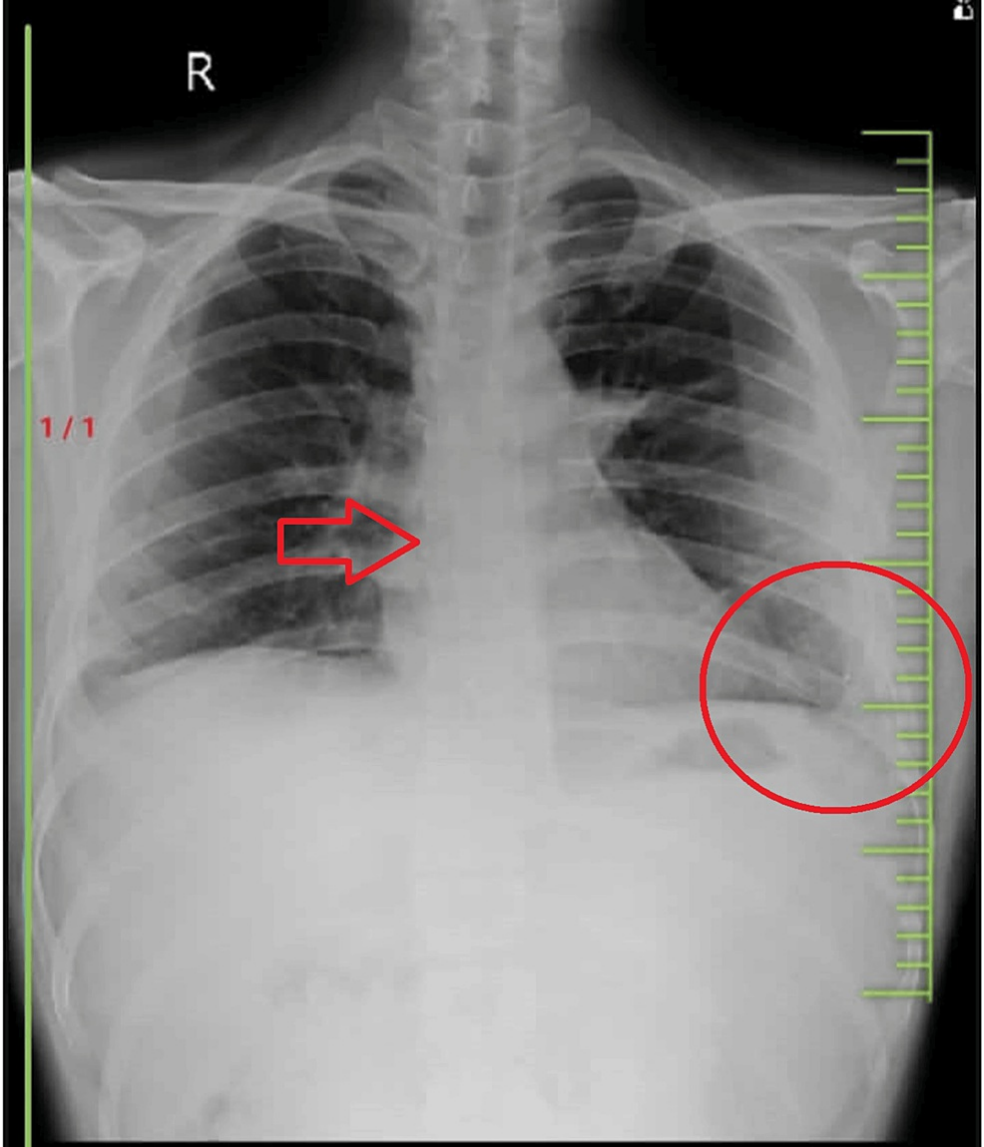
An anteroposterior view of the thorax X-ray shows an elevated angle, mild cardiomegaly, and mild cavitary lesions in the right middle and lower lung fields. The arrow shows the cardiomegaly, and the circle shows the cavitary lesion with bronchovesicular margins.
